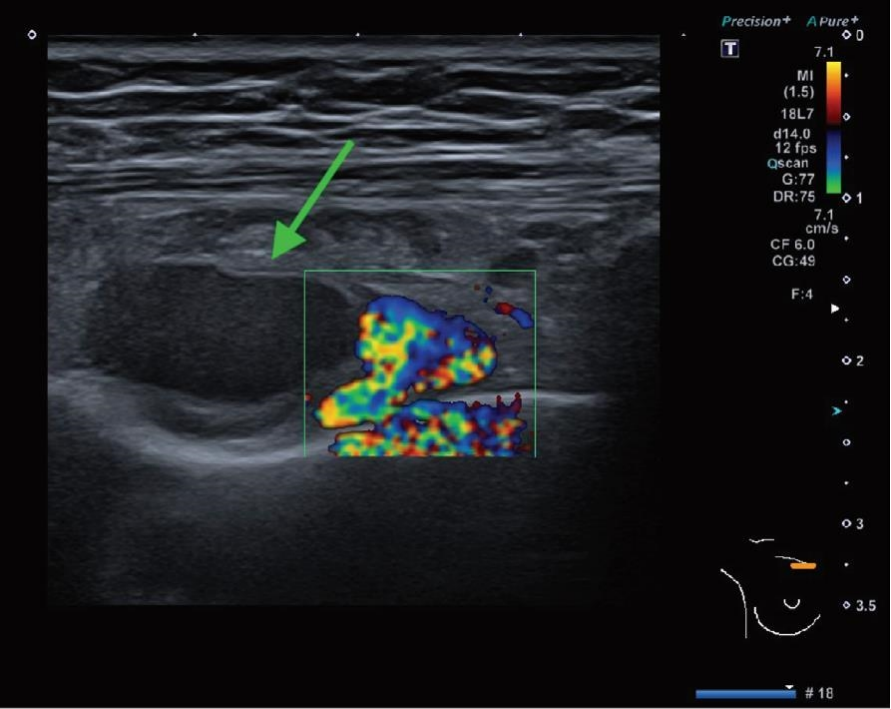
Breast ultrasound image (27/10/23) shows abnormal internal mammary chain node (green arrow).
